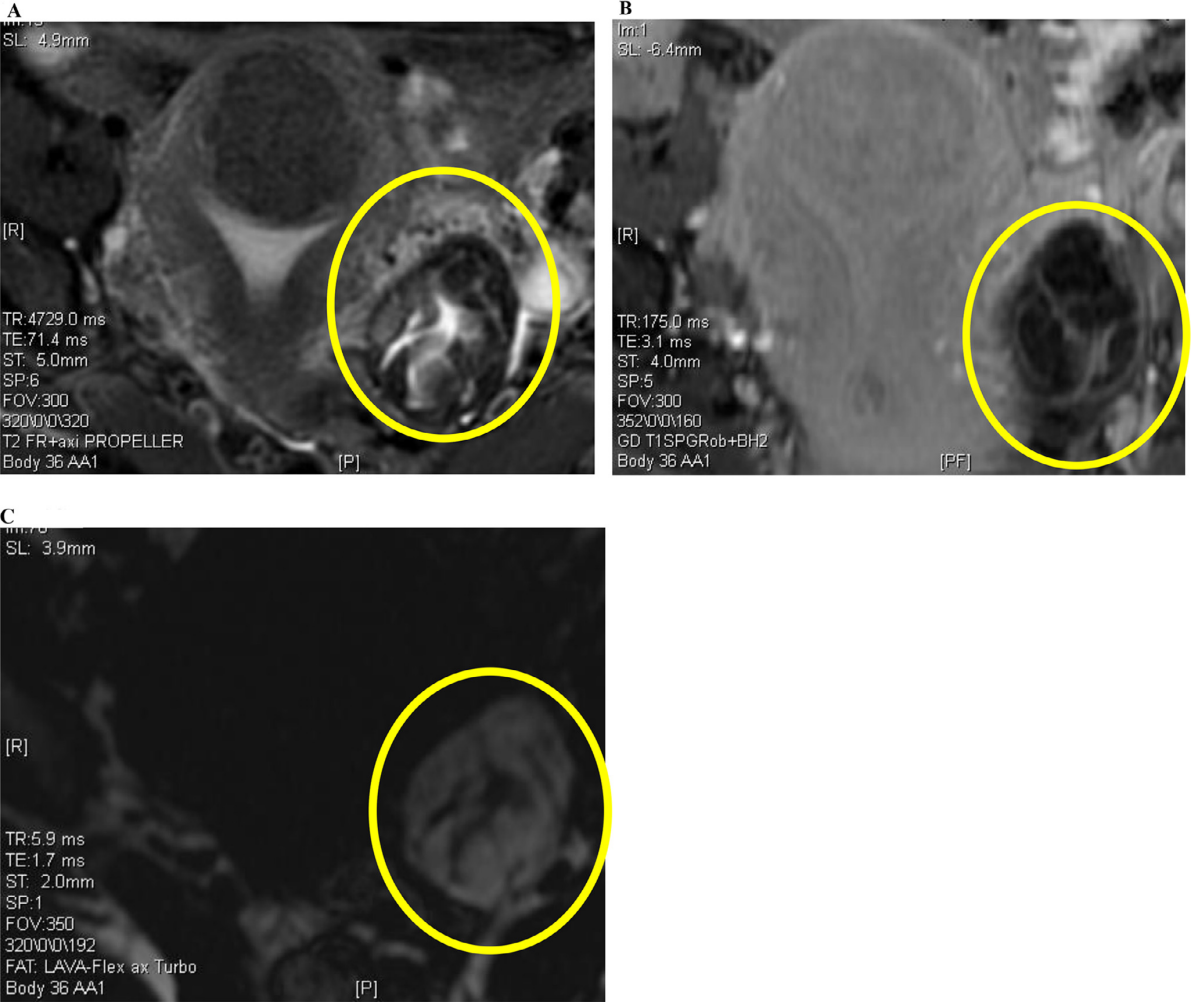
T2-weighted (A), T1-weighted (B), and fat-suppressed T1-weighted (C) images. Circled sigmoid mesenteric lipoma resembling an ovarian tumor.
